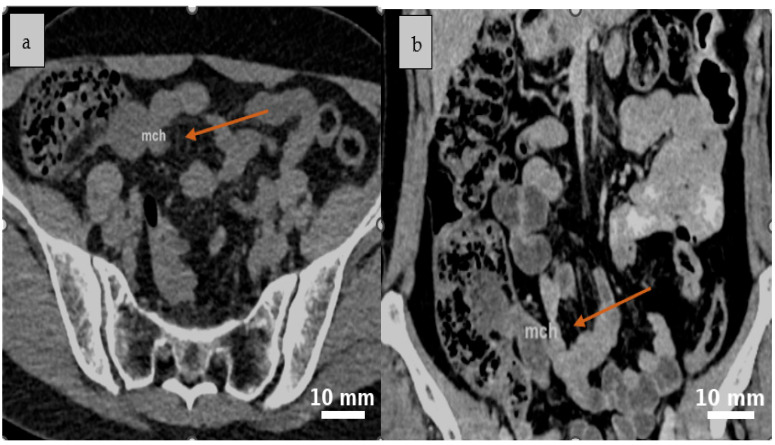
A well-defined, round to oval cystic lesion with fluid attenuation is identified in the ileocecal region on abdominal CT scan native (a) and confirmed on post-contrast coronal reconstruction, consistent with a mesenteric cyst (b). Yellow arrows indicate the cystic lesion (*mch = mesenteric cystic hygroma—lymphatic malformation*).
